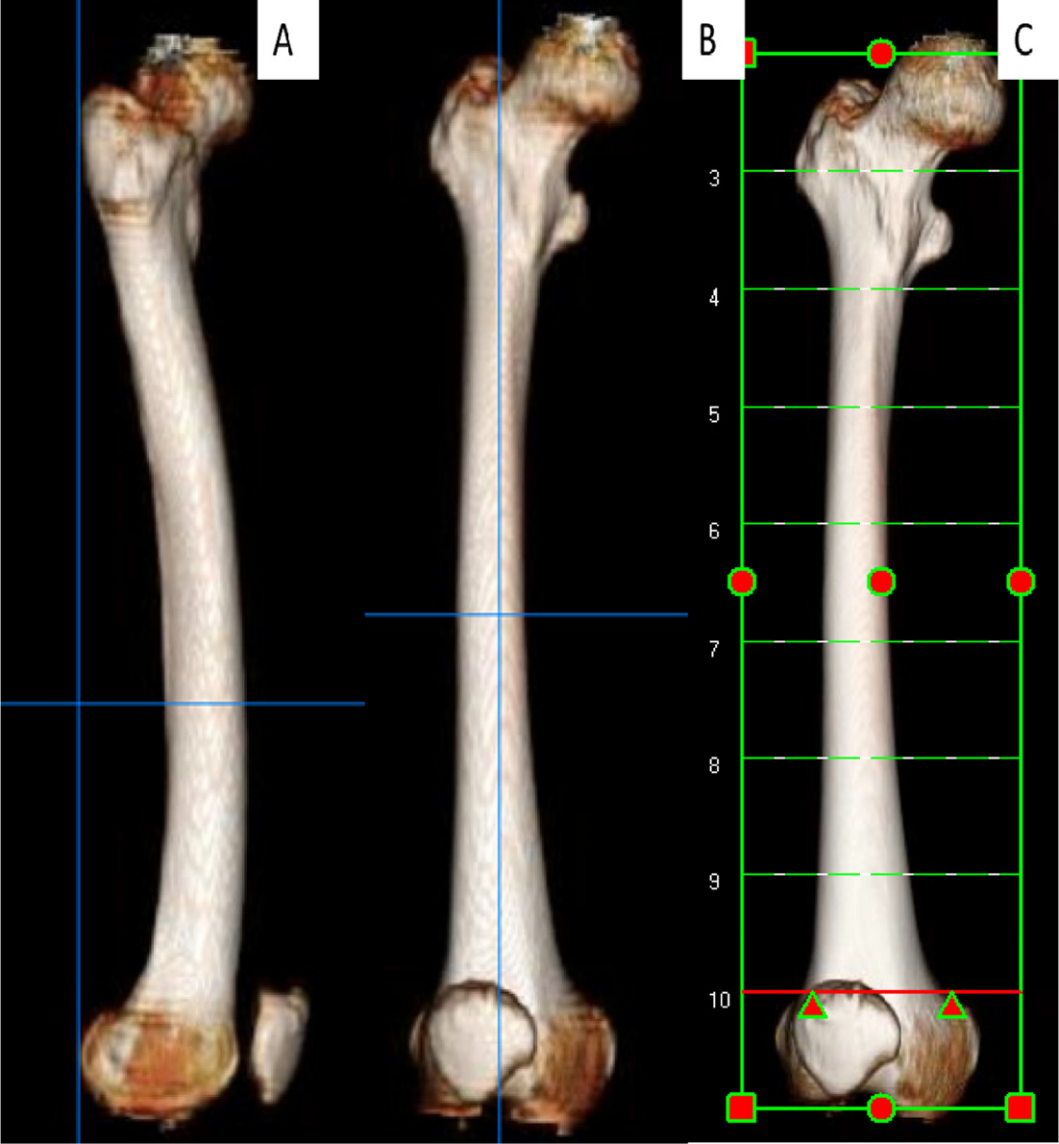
(A) Lateral view of the femur; both condyles are overlapped and a vertical line is tangential to the posterior condylar line and most posterior point of the greater trochanter, (B) anteroposterior view of the femur obtained by rotation of the lateral view 90°, (C) levels of the CT cuts starting at the widest intercondylar diameter and spaced at 5 cm intervals.
